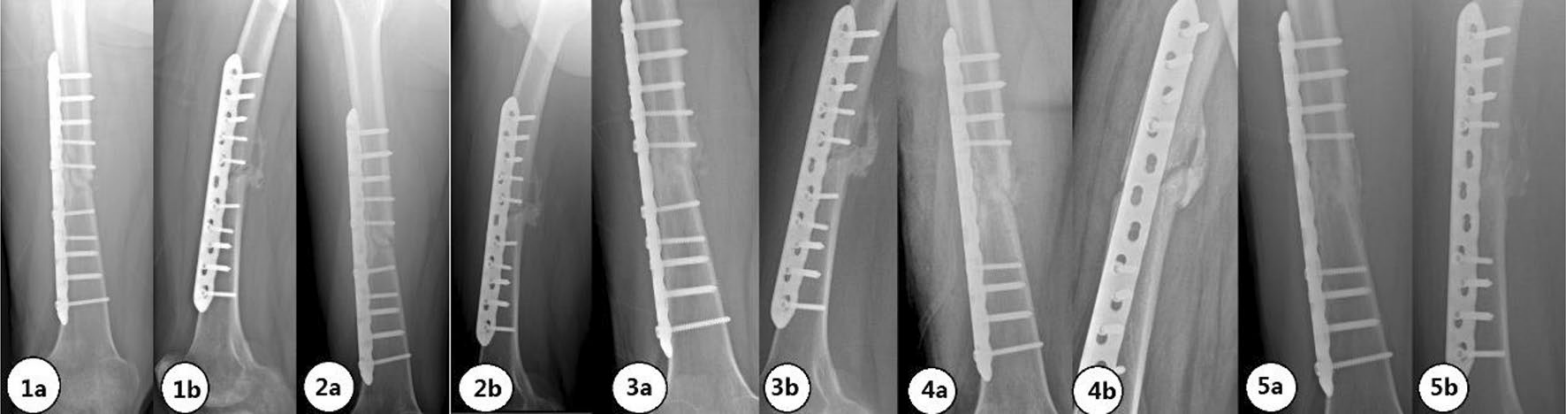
X-ray images at 6 (1), 7 (2), and 10 (3) months after the fixation operation, after bone grafting (4), and at 19 months after the fixation surgery (5). Anterior view (a). Lateral view (b).
